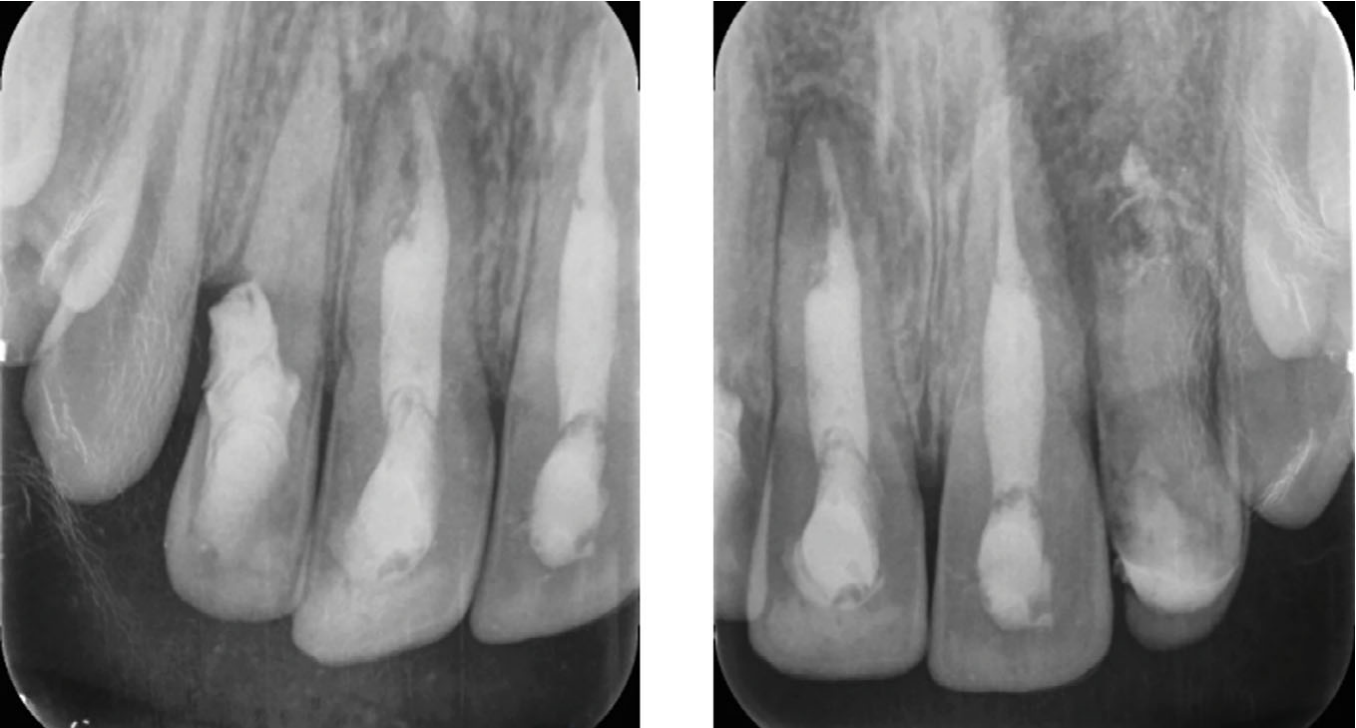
Intraoral periapical radiographs of 24 months' follow-up.
